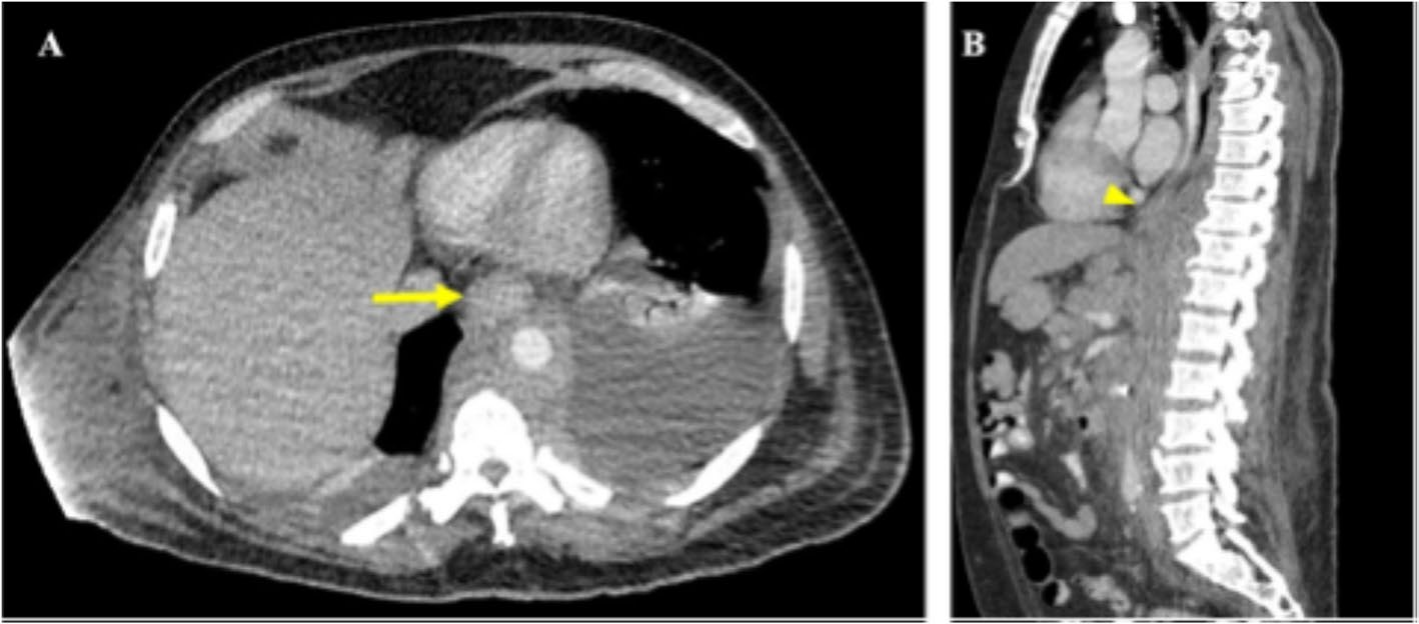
CT images of the chest/abdomen/pelvis with IV and oral contrast (A) Transverse section showing abnormal thickening of the distal esophagus (arrow). B Sagittal section showing abnormal thickening of the distal esophagus at the gastroesophageal junction (arrowhead)
